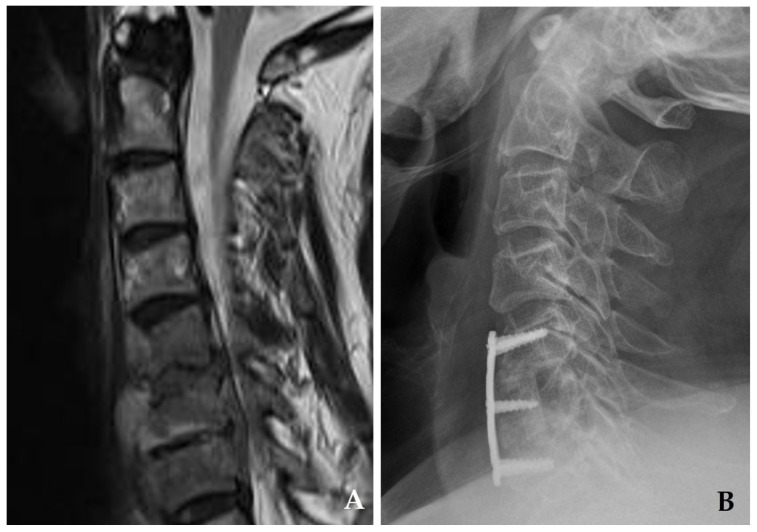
Initial magnetic resonance imaging (A) demonstrates disc space narrowing at C5–C6 and destructive changes in the C6 vertebral body with retropharyngeal and epidural abscesses. The patient underwent C5–C7 corpectomy with autograft and anterior plating (B), achieving solid fusion and complete resolution of spondylodiscitis at six months postoperatively.
